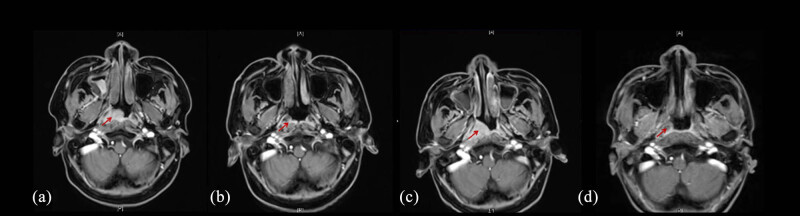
MRI scans before and after treatment. (a) The arrow shows a soft tissue mass at the right pharyngeal recess (2021.5.3). (b) The arrow shows that the mass significantly shank at 1 month posttranspantation (2021.7.8). (c) The arrow shows that the mass enlarged again at 1.5 months posttranspantation. (d) The arrow shows that the mass was disappeared at 11.5 months post-transplantation (6 months after immunoradiotherapy).
